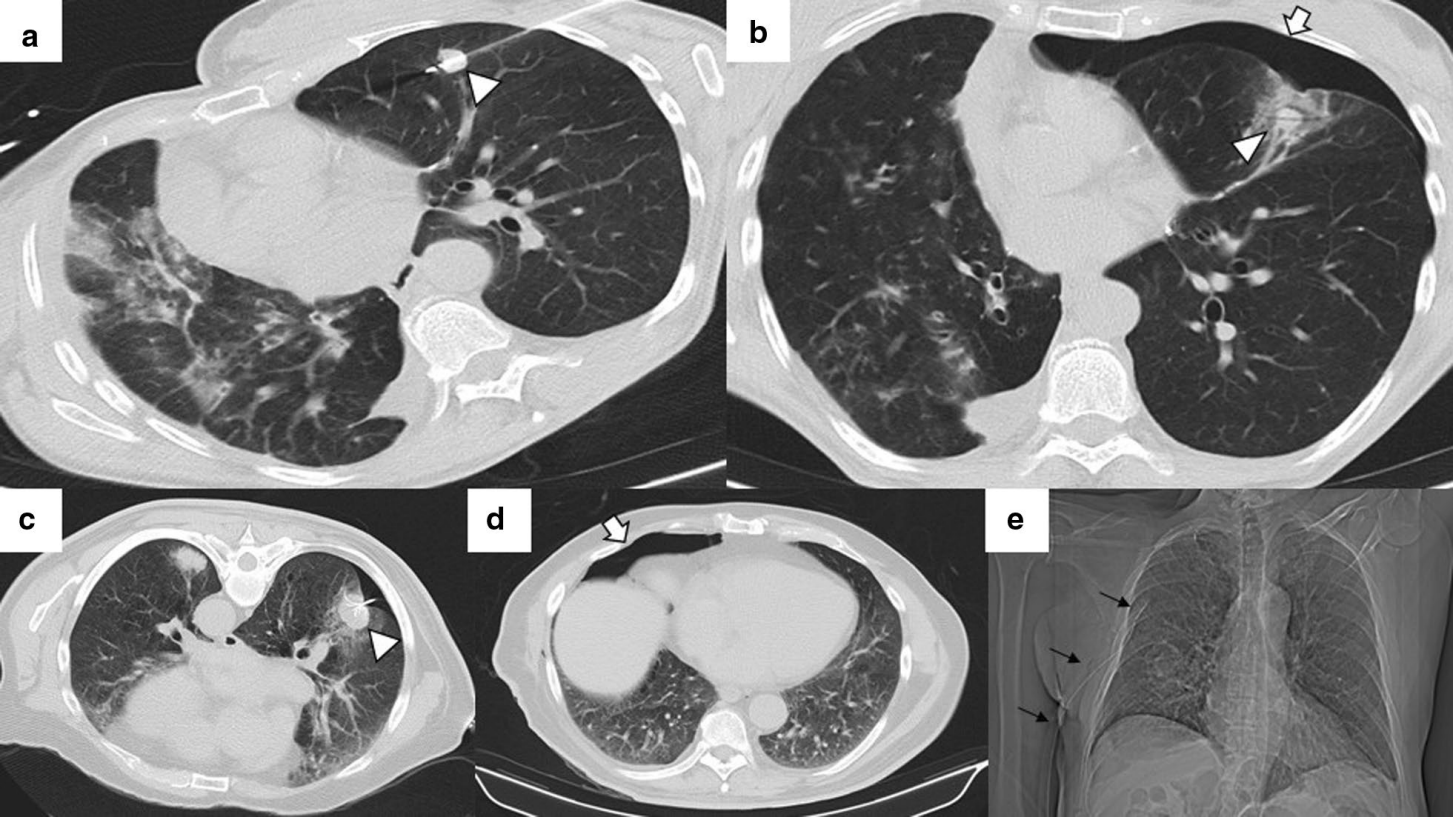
Mild pneumothorax after image-guided percutaneous lung ablation. a, b CT-guided MWA in a single lung metastasis from colon cancer in the upper left lobe. Note the correct probe position in the center of the lesion (triangle). In the CT performed immediately after the procedure, the patient presented a mild pneumothorax (arrow). The pneumothorax was resolved spontaneously without the need for a chest tube. Note the peripheral ground-glass halo with a central consolidation (triangle in b), which indicates a correct treatment. c–e CT-guided RFA lung ablation in a lung metastasis from a basal cell skin carcinoma. The expandable probe correctly englobes the lesion (triangle in c). In this patient, a pneumothorax was also observed immediately after the ablation. (arrow in d). Although pneumothorax is similar to in A-B, the patient's clinical situation required a chest tube (black arrows in e)
